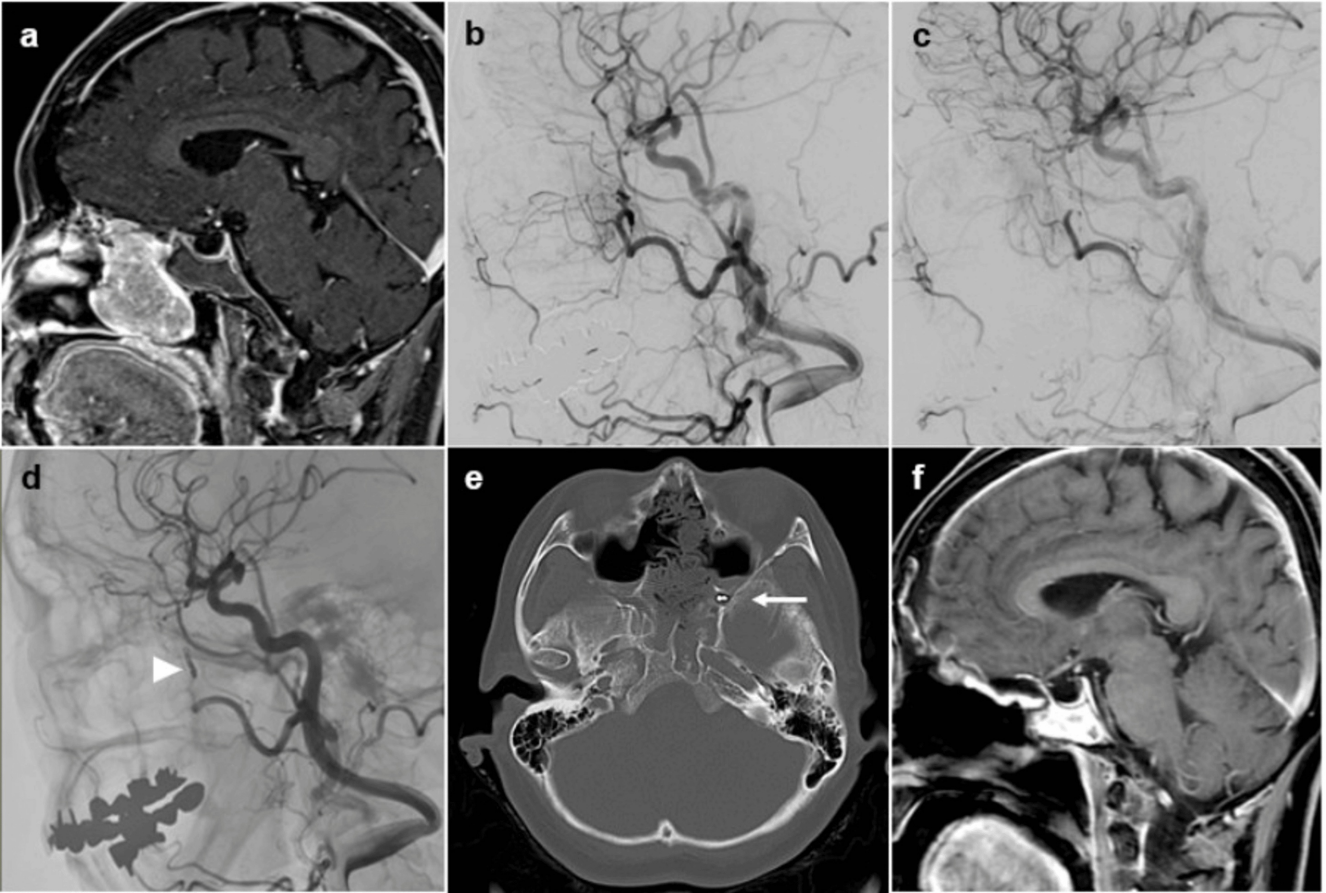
Pre- and postoperative multimodal imaging of Case 1 (esthesioneuroblastoma). (a-f) Preoperative MR image and angiography demonstrate hypervascularity of the tumor supplied by the sphenopalatine artery(a, b). Post-embolization angiography confirms disappearance of the tumor blush(c). Post-embolization and postoperative images show complete devascularization and coil placement at the feeding branch (arrowhead in d and arrow in e).
